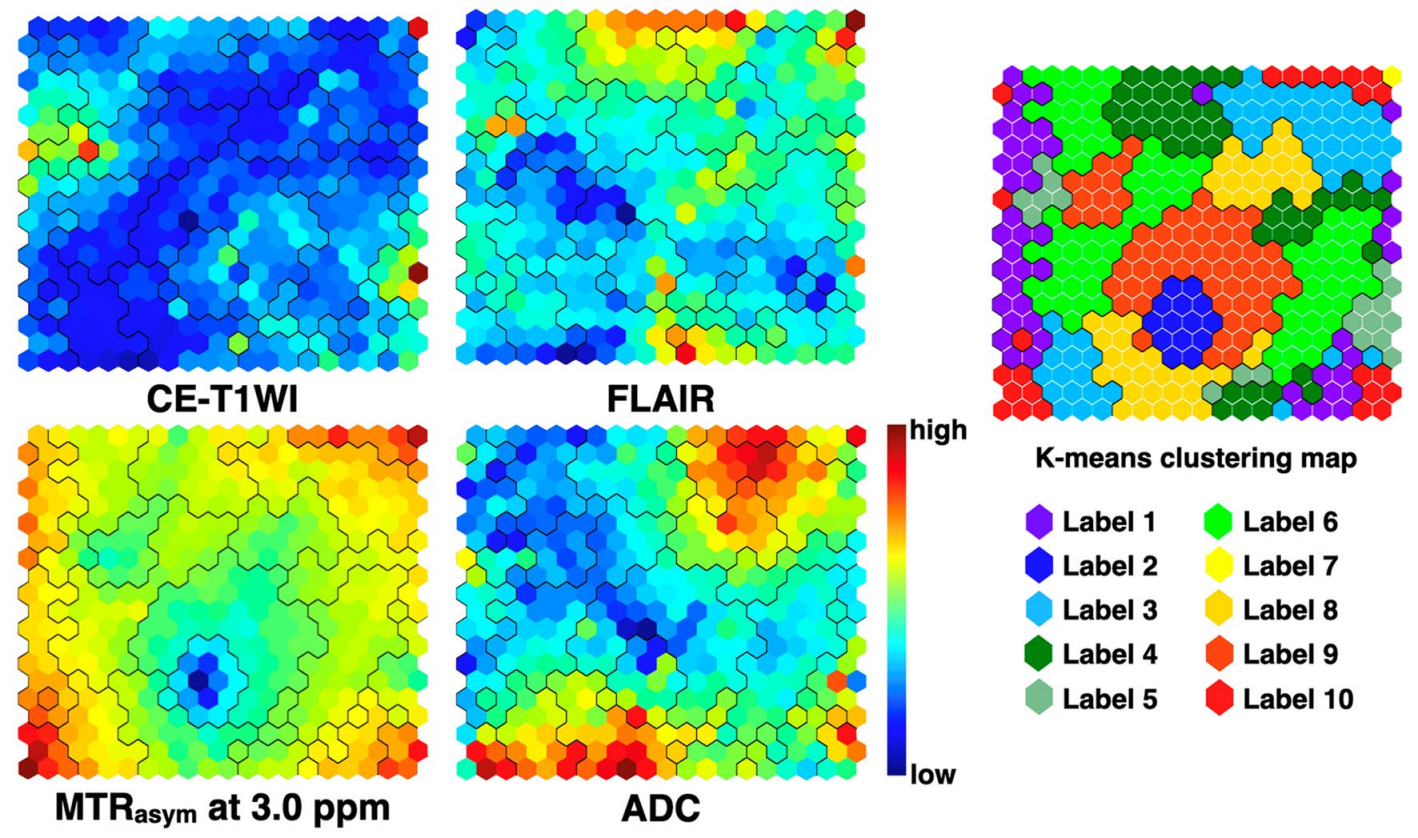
Component planes with a SOM for CE-T1WI, FLAIR, MTR_asym_ at 3.0 ppm, and ADC colorized from blue to red according to each value, with red indicating a higher weight. The inter-class borderlines obtained by K-means clustering with K = 10 are shown on the SOM component planes as black lines between the nodes. Detailed profiles can be seen on the K-means clustering map from labels 1 to 10 shown at the far right.
